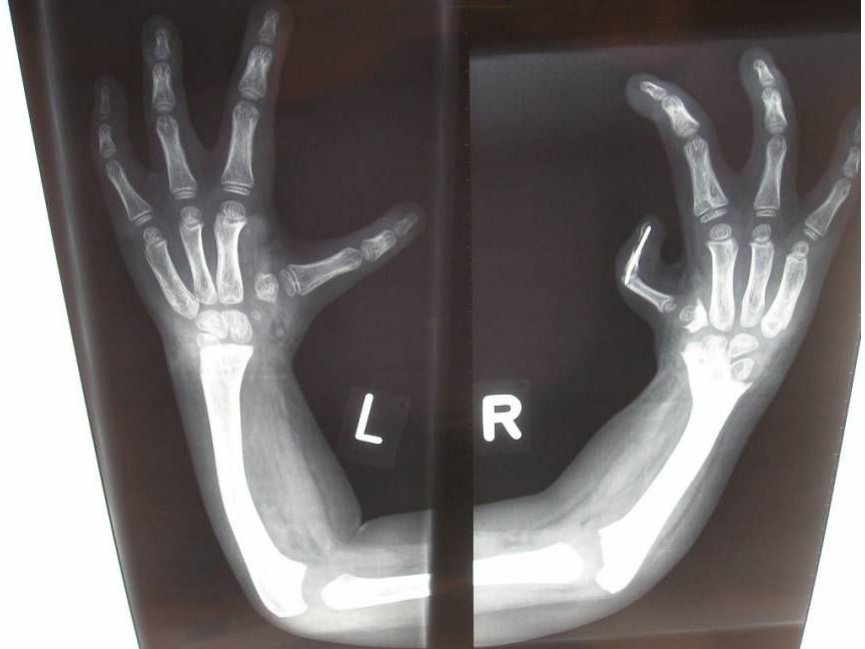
Anteroposterior radiograph of the upper limbs after the reconstruction showed profound radial aplasia, metaphyseal cupping associated with hypoplastic/defective ossification of the carpal bones. Pseudoepiphyses with proximally situated newly reconstructed thumb. The patient had reconstruction of the thumbs with pollizisation of the second finger. This means a rotation-osteotomy of the second finger to the thumb position with reconstruction of the commissural between the new reconstructed thumbs and the second finger, which was originally the third finger.
